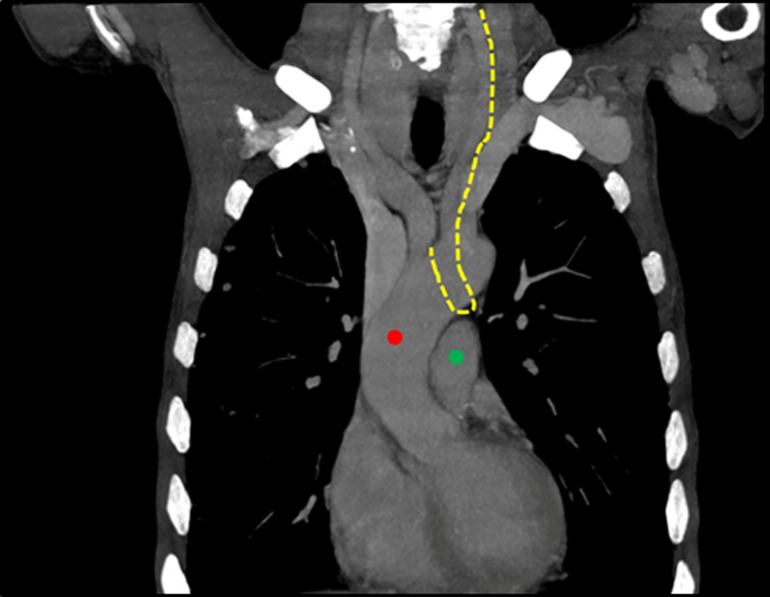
normal chest computed tomography scan showing the presumed trajectory of the left recurrent laryngeal nerve (yellow ling) as it passes through the aortopulmonary window between the thoracic aorta (red circle) and the pulmonary artery (green circle)
